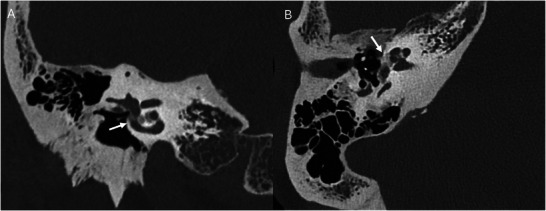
Photon‐counting detector computed tomography (PCD CT) images of otospongiosis. Coronal (A) and axial (B) PCD CT images showing lucency in the otic capsule involving the right fissula ante fenestram, oval window, cochlea, vestibule, and facial nerve canal compatible with otospongiosis (arrow).
